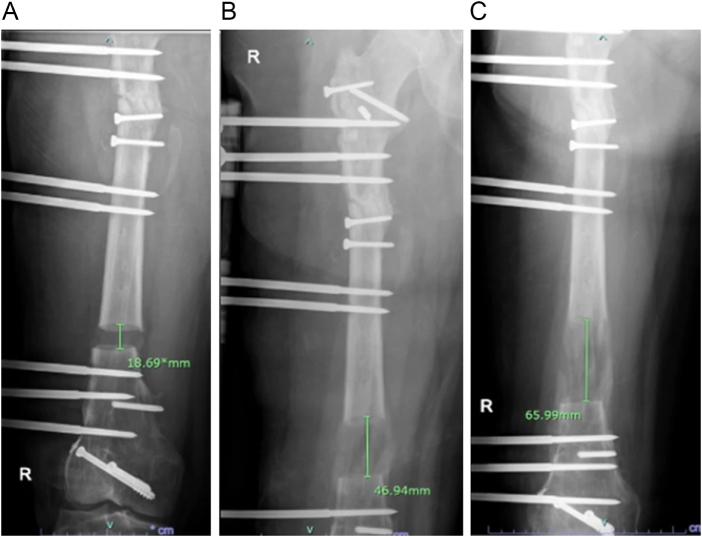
(A) Radiograph at 1-month post-op; (B) Radiograph at 3 months post-op; (C) Radiograph at 5 months post-op.
